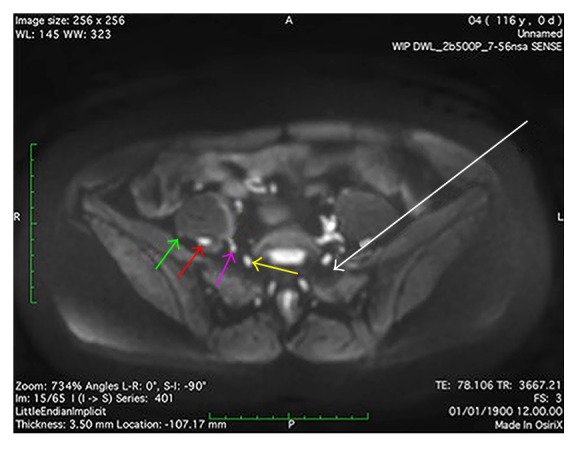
Axial diffusion weighted MRI at the level of the cranial border of the sacral ala, demonstrating a possible anterior approach to the lumbosacral plexus in the supine position. A needle (white arrow) can be inserted close to the anterior superior iliac spine and advanced between the psoas major and iliacus muscles. A first injection of local anesthetic into the* retropsoas subcompartment* will spread to the femoral nerve (red arrow) and the lateral femoral cutaneous nerve (green arrow). A second injection of local anesthetic into the* parapsoas compartment* will spread to the anterior rami of spinal nerves L4 and L5, the lumbosacral trunk (yellow arrow) and the obturator nerve (pink arrow).
